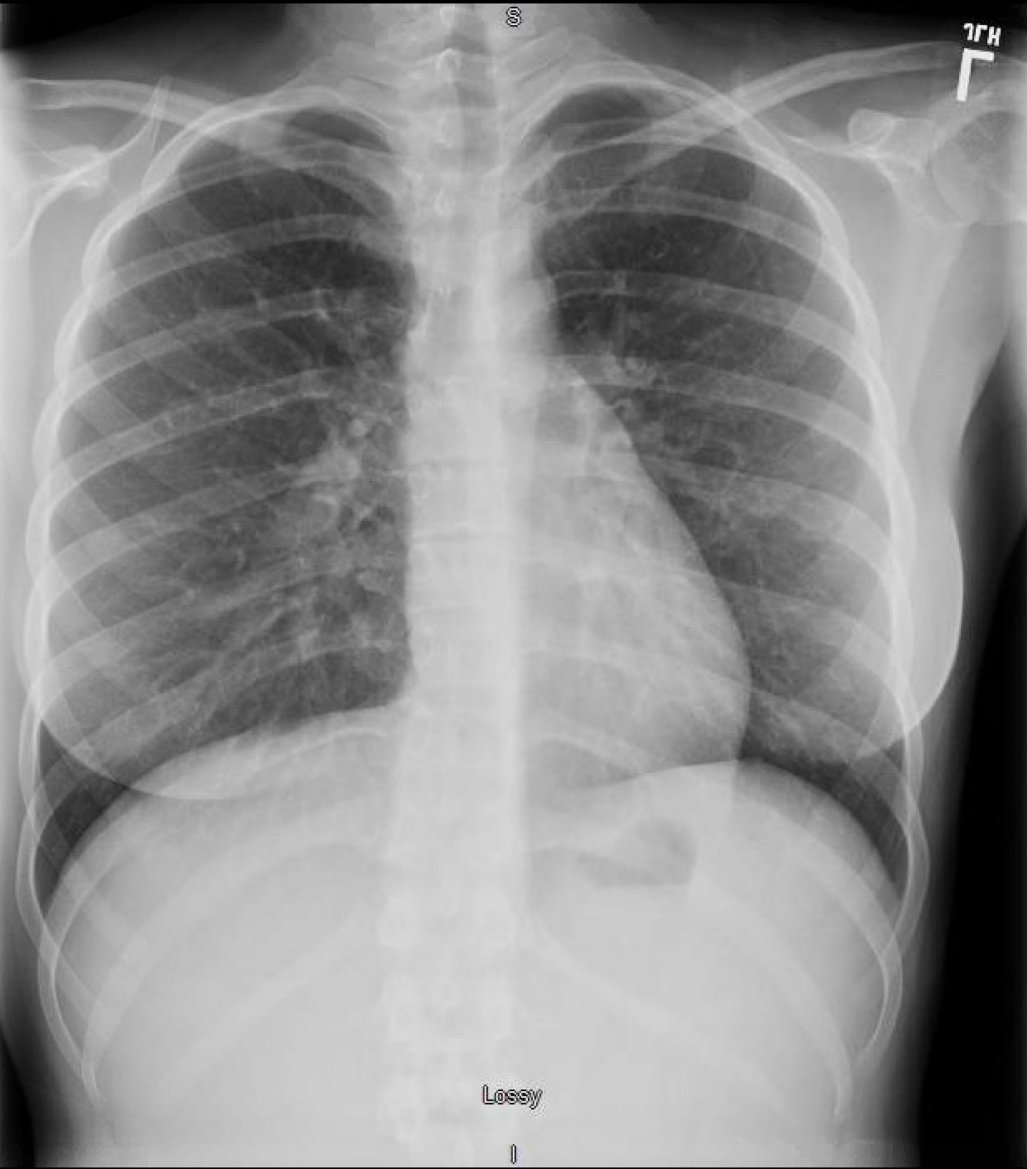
Chest X-ray (anteroposterior view) showing normal findings.
